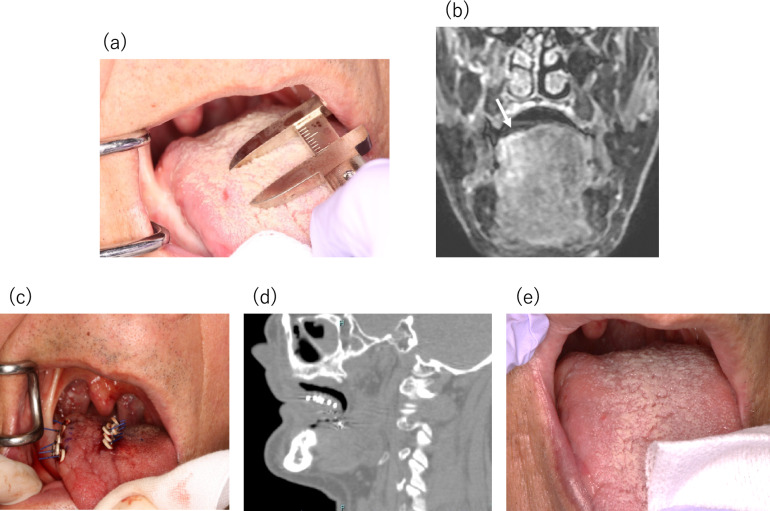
Clinical course of Patient No. 5 with recurrent tongue SCC after brachytherapy treated with DaRT: a A tumor before treatment with size of 10 × 6 × 3 mm; b MRI coronal section showing tumor (arrow); c State of six DaRT seeds implanted in a single plane; d Sagittal reconstruction CT image of inserted DaRT seeds; e No change was observed until 4 weeks after initiation of treatment, but the tumor disappeared after 10 weeks of treatment
